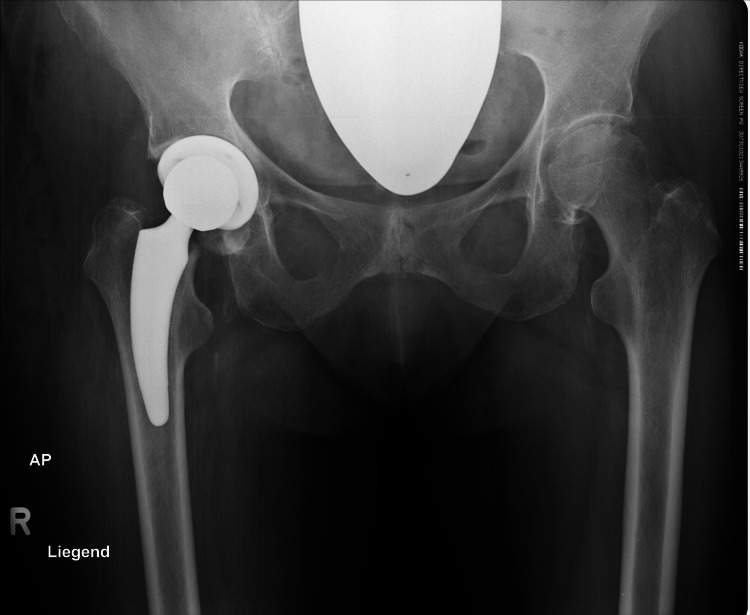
Postoperative radiographs after implantation of an Optimys stem (Mathys Ltd., Bettlach, Switzerland)
